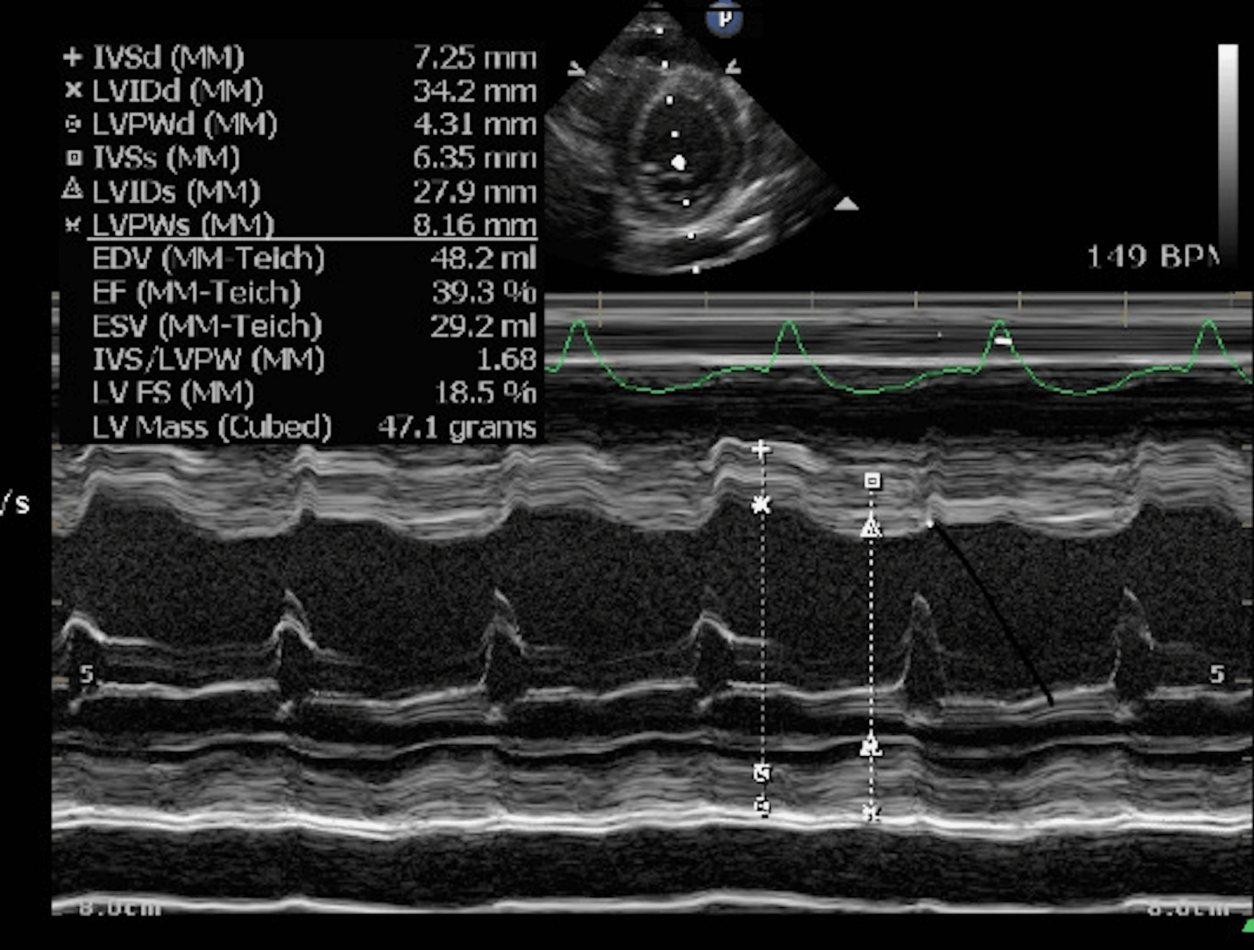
A cardiologist-performed echocardiogram A cardiologist-performed echocardiogram demonstrated a moderately reduced left ventricular systolic function with an ejection fraction (EF) of 39%.
